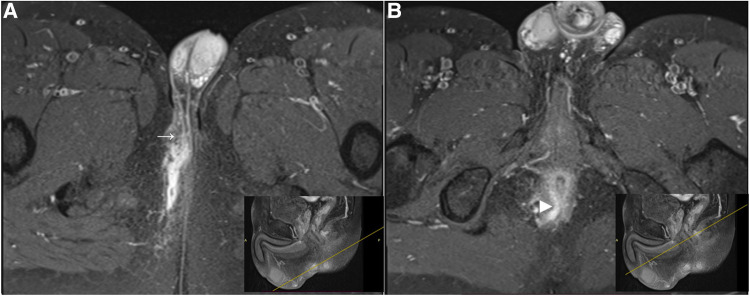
A high trannsphincteric fistula extending into the right scrotum. Post-contrast FS axial T1-weighted images show (A) an anal fistula extending into the right scrotum (arrow) with a curved track (B) which opens into the posterior wall of the anal canal at 6 o'clock position (arrowhead). The corresponding location (yellow line) where the fistula penetrates the external sphincter. The fistula traverses the upper half of the anal canal and we classified it as a high transsphincteric fistula.
